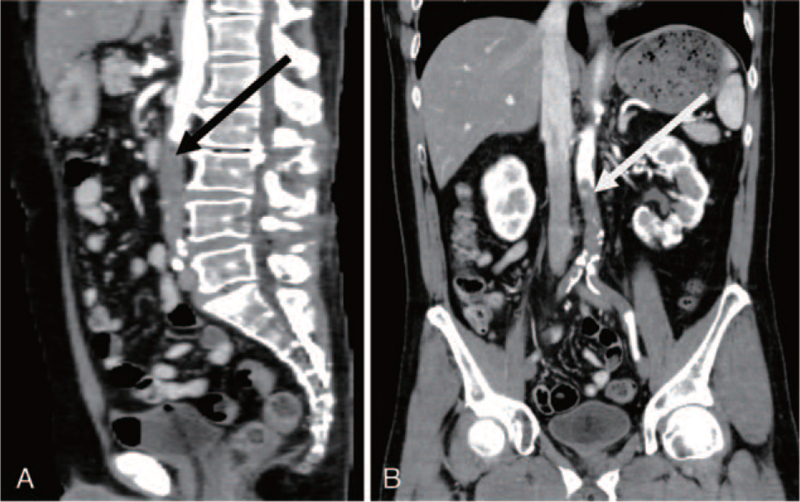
Visualized thrombus within abdominal aorta (arrow) on CT angiogram image of a patient with acute aortic occlusion. (A) Sagittal view of CT angiogram. (B) Coronal view of CT angiogram.
